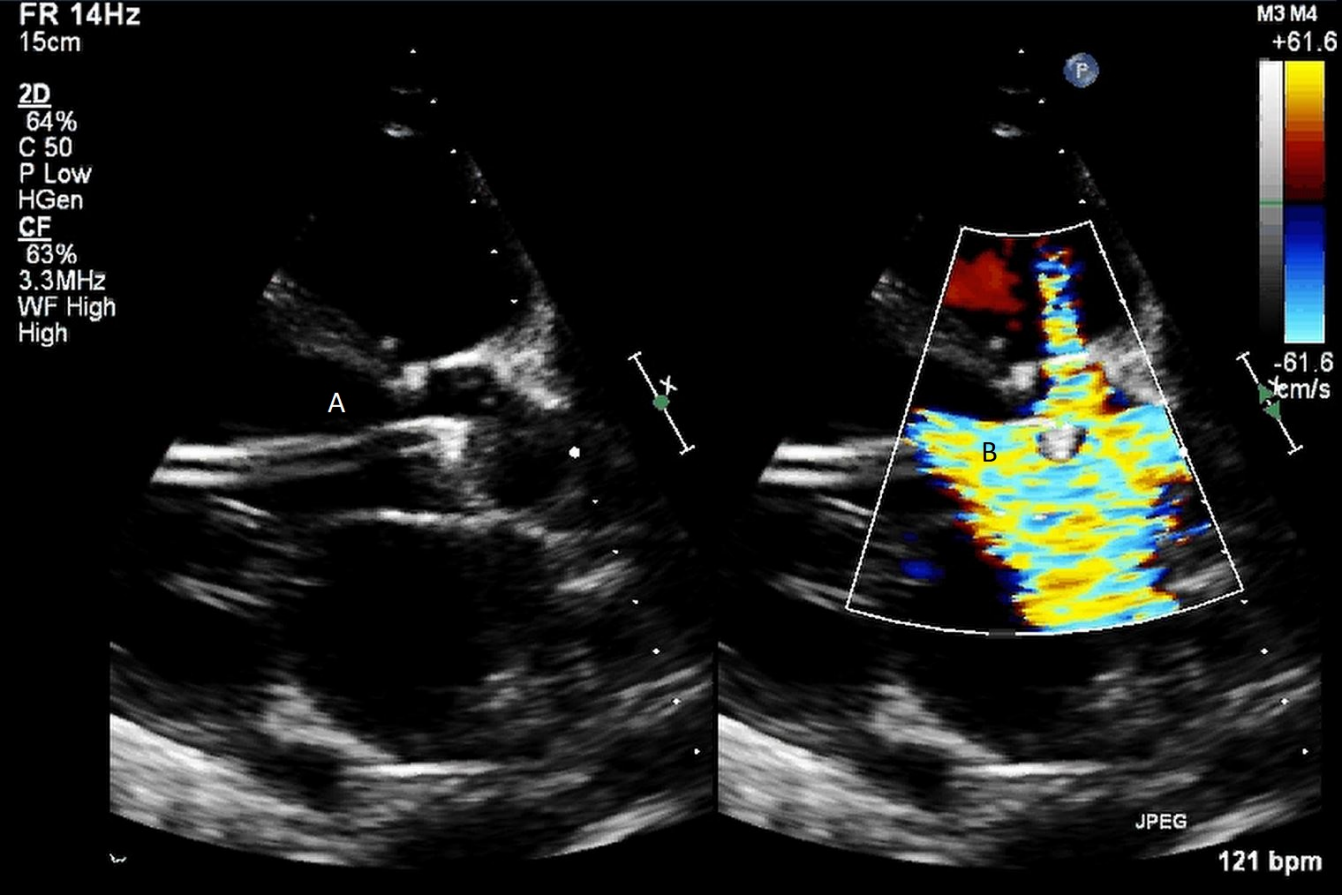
Impella (A) visualized on parasternal long axis view on TTE. Color flow imaging shows that the impella outlet is too low (B) as the mosaic
